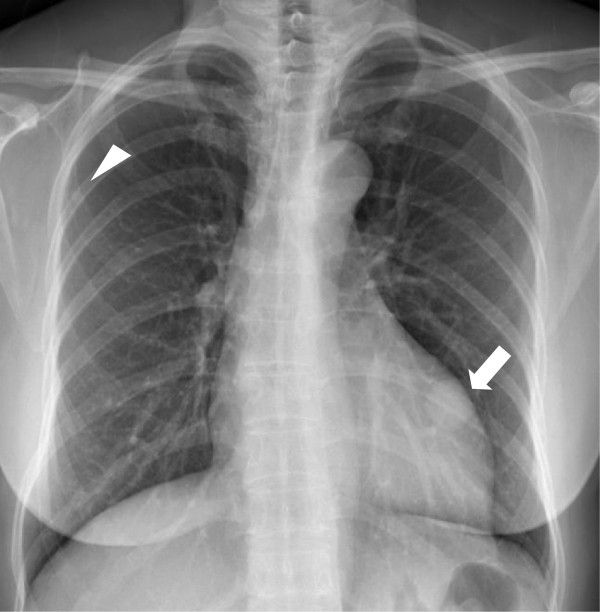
Chest radiograph. There is a 2.6-cm well-defined oval mass in the left lower lung (white arrow) and a small ovoid nodule in the right upper lobe field (arrowhead).
